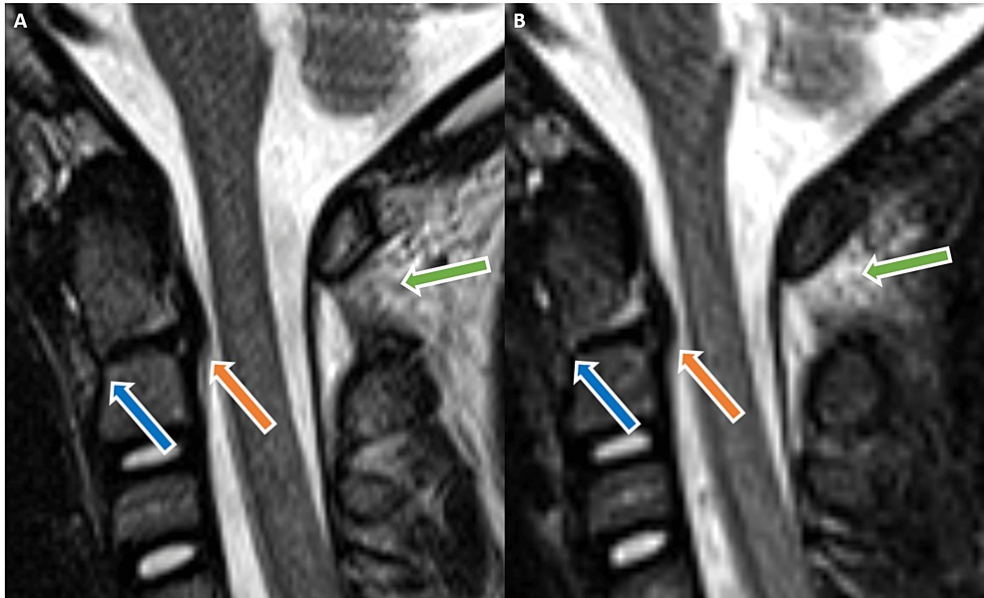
Sagittal T2-weighted (A) and STIR (B) MR imaging of the cervical spine demonstrates anterior subluxation of the dens with surrounding fluid (blue arrows). There is mild indentation of the ventral thecal sac secondary to truncated posterior longitudinal ligament (orange arrows). Widening of the posterior atlantoaxial space with associated hyperintensity is also noted (green arrows). STIR - short tau inversion recovery, MR - magnetic resonance
